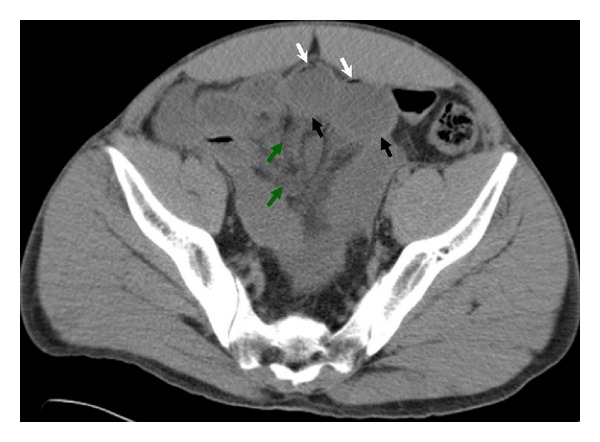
Computed tomography of the abdomen reveals multiple loops of mid and distal small bowel were dilated (black arrows) with pneumatosis intestinalis. The white arrows mark areas of air in the small bowel wall. The surrounding mesentery is edematous as marked with the green arrows.
